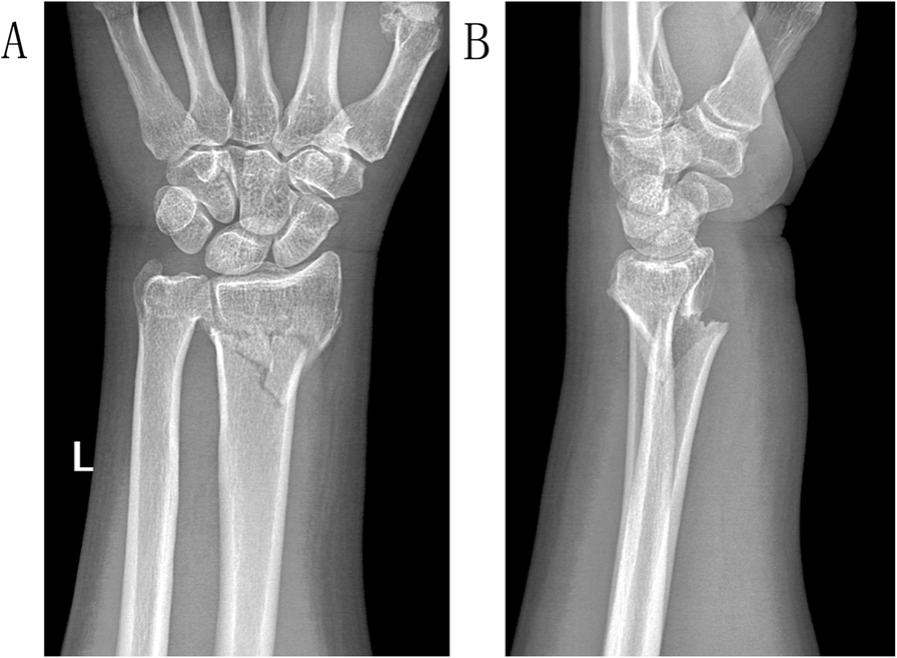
A 65-year-old male patient suffered a trauma to the left wrist when he slipped and fell. The frontal and lateral X-ray films showed distal radius fracture with reduced radial height (11.06mm), radial inclination (19.88°), and volar tilt (−18.80°). The radial shift (+2.37mm) and ulnar variance (+1.74mm) were abnormal as well. AO/OTA classification was A_2_ type
